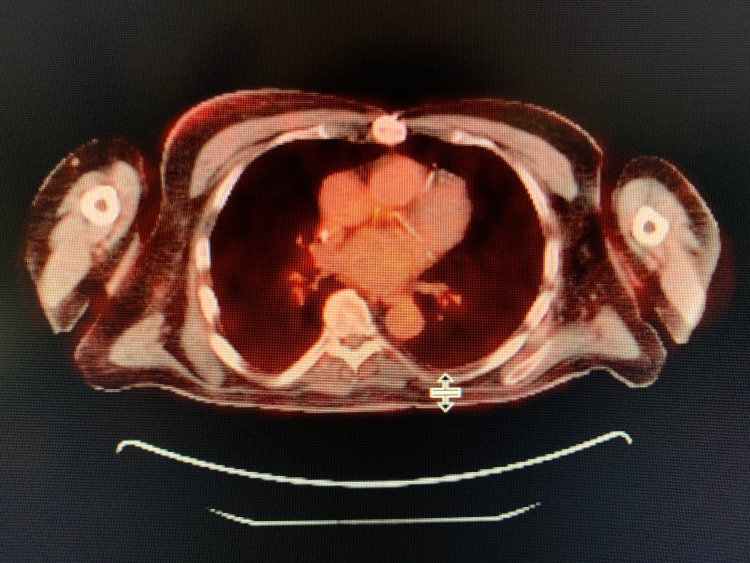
PET scan showing no SUV/FDG uptake in the hilar region SUV, standardized uptake value; FDG, fluorodeoxyglucose
